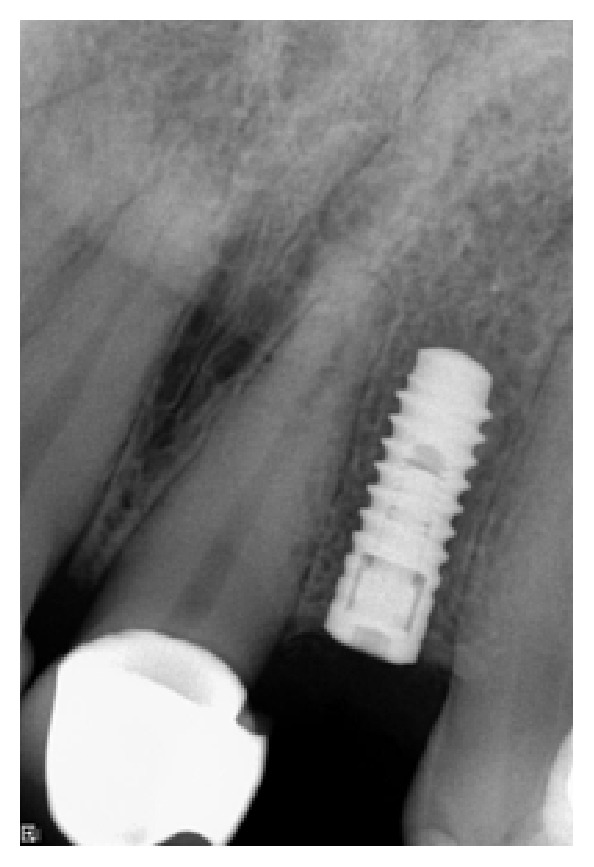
Periapical radiograph following implant placement.
